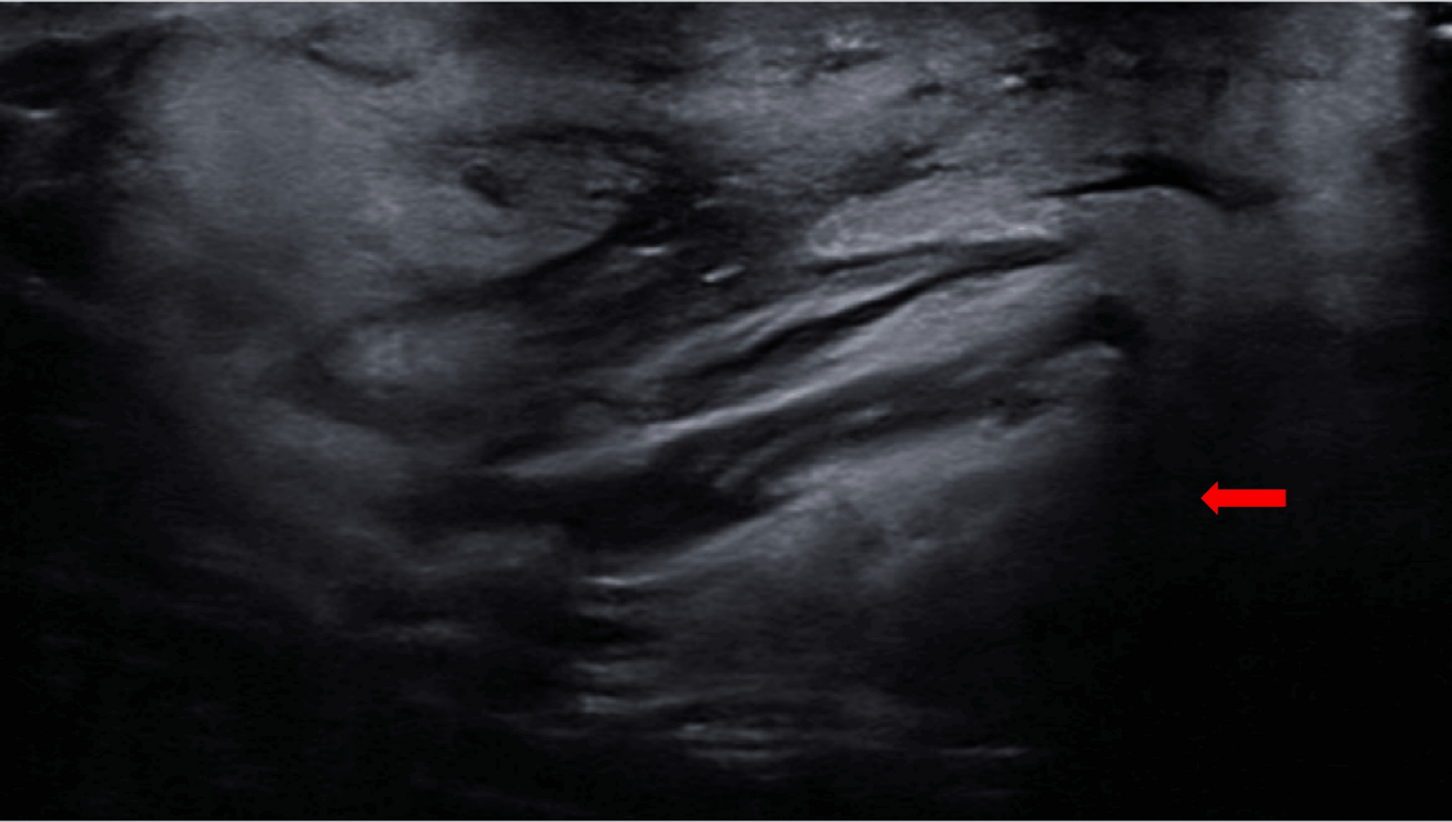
Initial ultrasound image of the left SCL mass Red arrow: SCL visualized via the ultrasound in a sagittal lateral view SCL: Spindle cell lipoma
